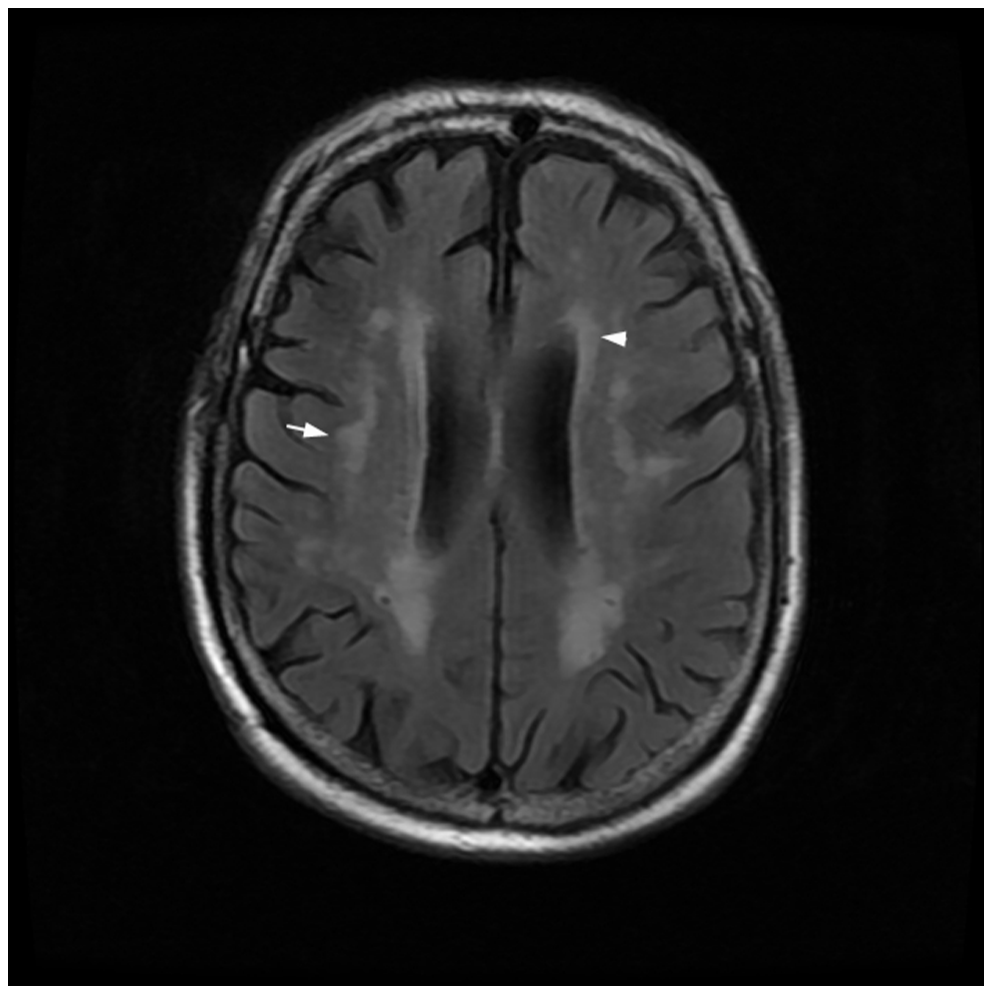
White matter hyperintensities on T2-fluid-attenuated inversion recovery sequence (T2-FLAIR). Deep white matter hyperintensities (DWMH) and peri-ventricular hyperintensities (PVH) was indicated by arrow and arrow head, respectively.
